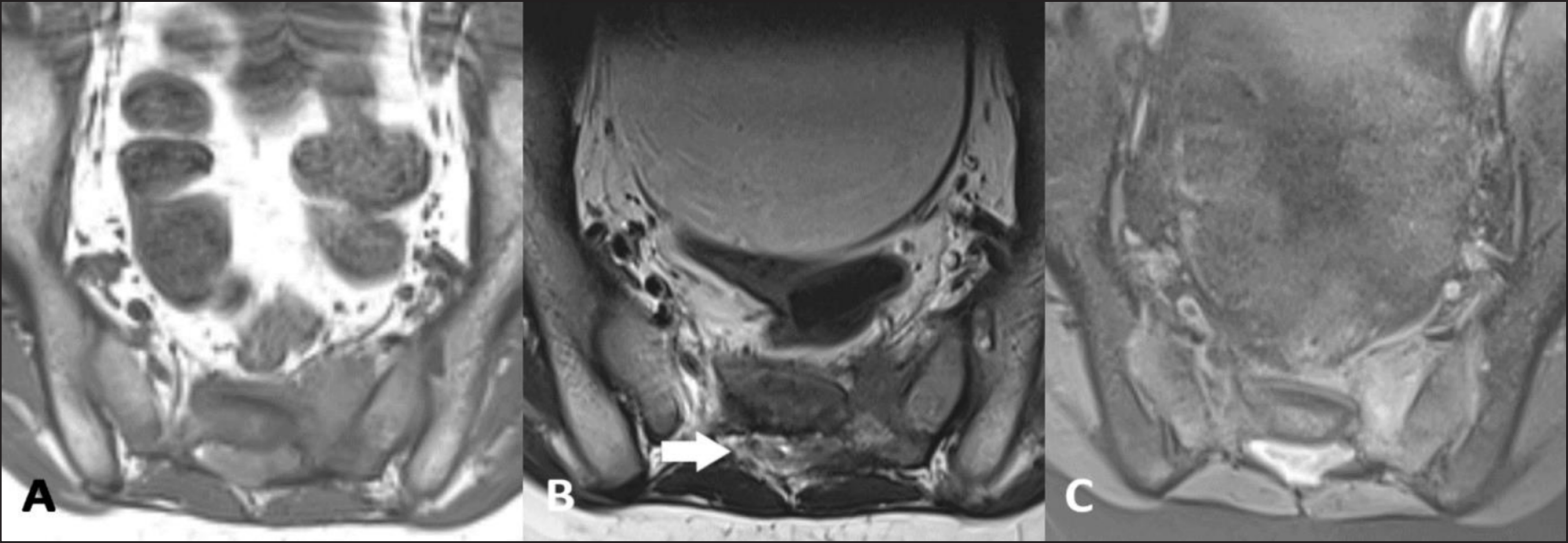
A 14-year-old male with sacral Ewing sarcoma. (a) Axial T1-weighted MR image shows a hypointense mass arising from left side of the sacral body. (b) Axial T2-weighted MR image shows a large soft-tissue mass, invading into the spinal canal (arrow) and presacral area. Note the absence of hyperintense cerebrospinal fluid signal. (c) Axial fat saturated post-contrast T1-weighted MR image shows enhancement of the tumour and the intraspinal component.
